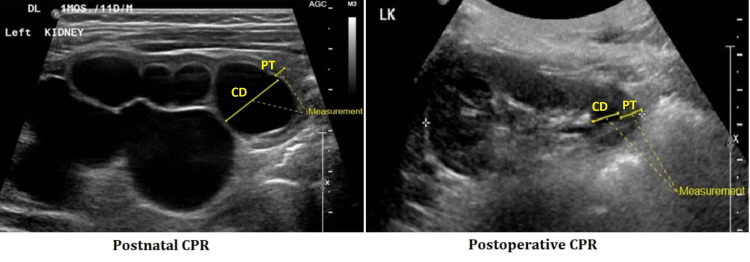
Measuring the CPR Sagittal ultrasonographic image of hydronephrotic kidney representing the measurement of CD and PT to calculate postnatal CPR and CPR after pyeloplasty for the same patient CPR: calyceal-to-parenchymal ratio, CD: calyceal diameter, PT: parenchymal thickness
